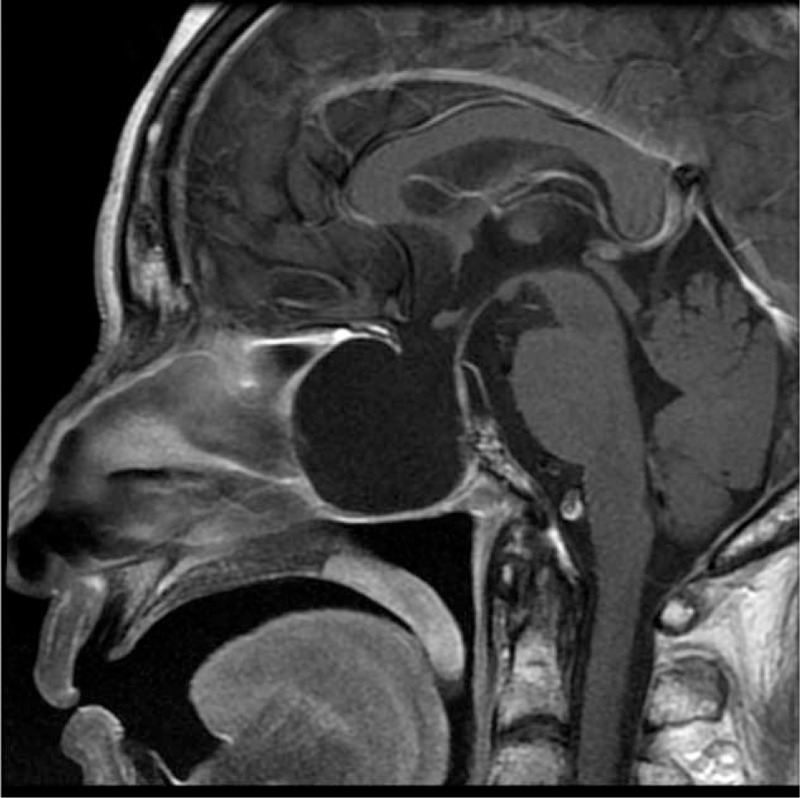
Empty sella combined with intrasphenoidal meningocele formation in magnetic resonance imaging (MRI) scan of brain. Sagittal T1-weighted. Huge cystic structure from pituitary sella herniated into sphenoid sinuses causing sellar floor destruction and wall of sphenoid and ethmoid sinuses remodeling is found.
